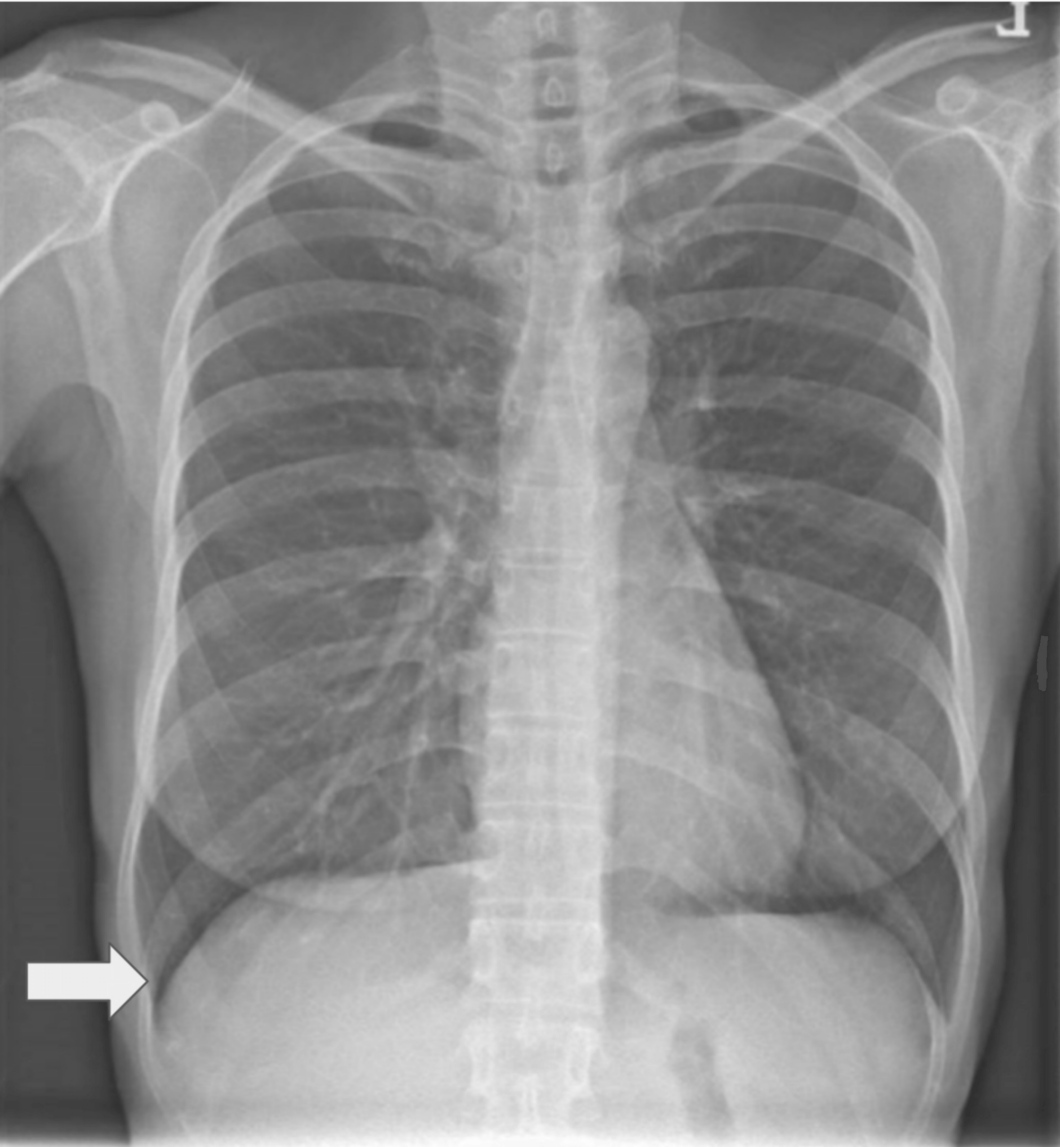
Erect chest X-ray The cardiomediastinal silhouette is normal. There is no focal lung consolidation or pleural effusion seen. The arrow points to sharp diaphragmatic borders.
